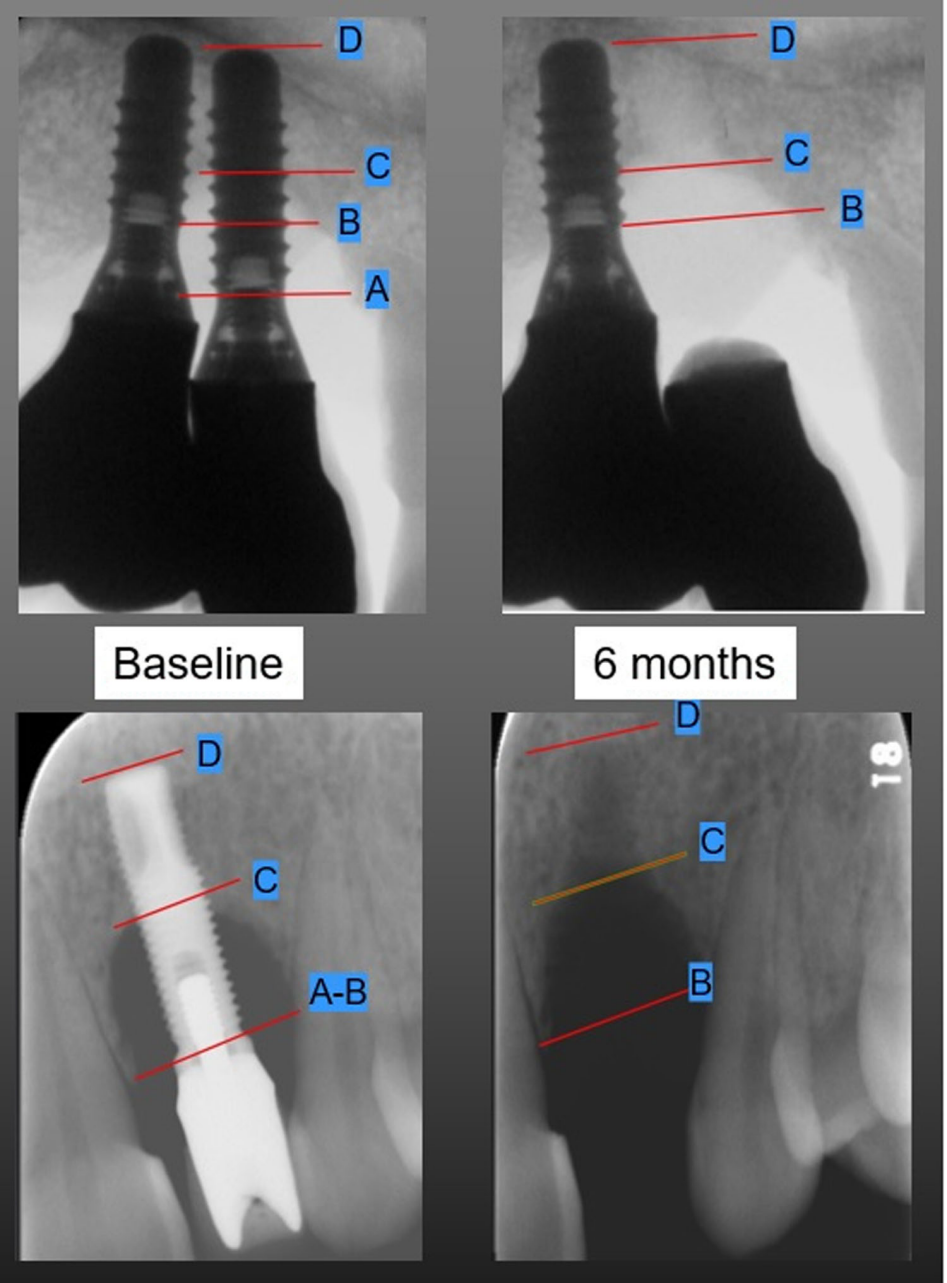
Radiographs before and after implant explantation: It is possible to note the different degree of mineralization in the apical portion between the alveola bony walls and the new formed bone.
